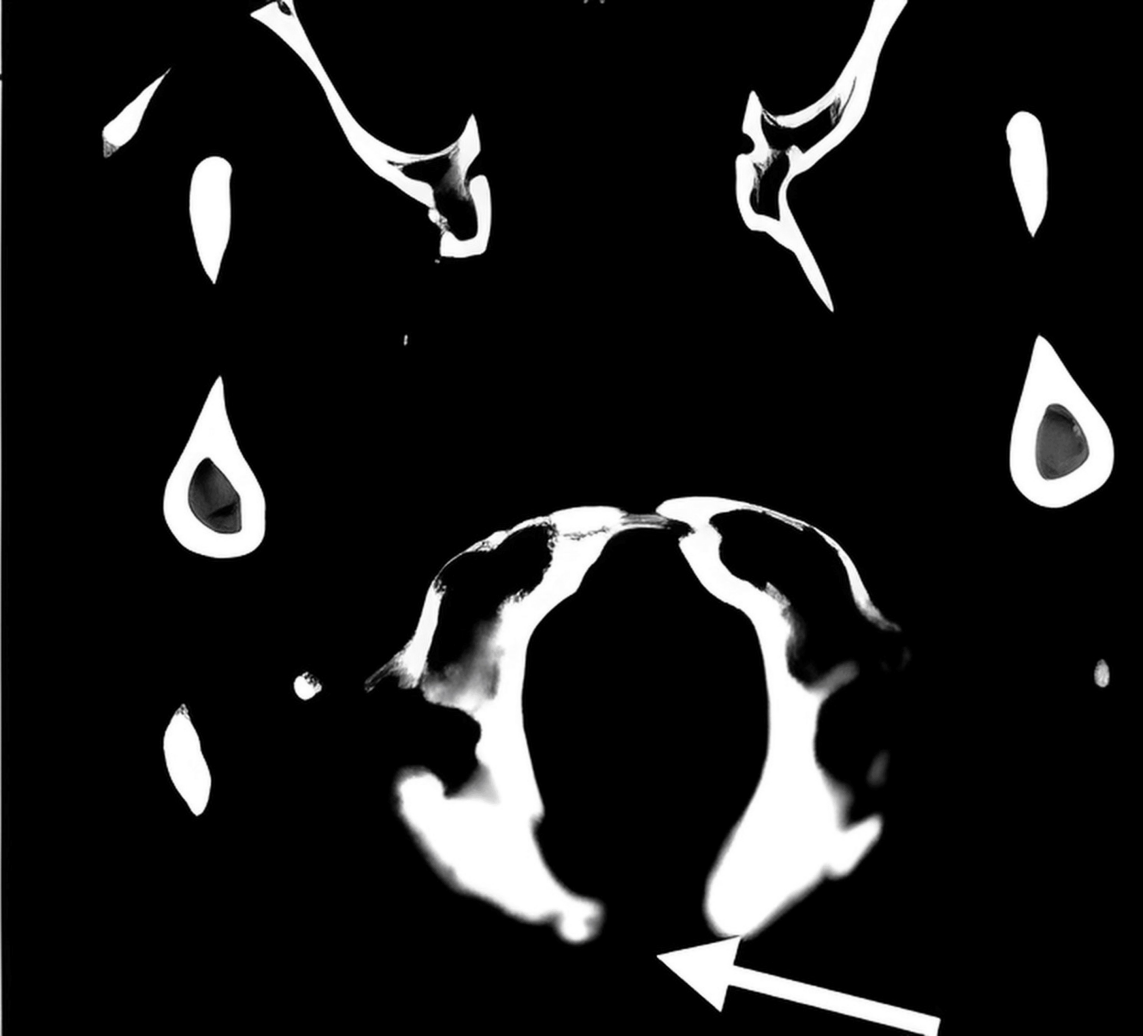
Postoperative CT images show the partial resection of the posterior arch of the atlas (white arrow).
